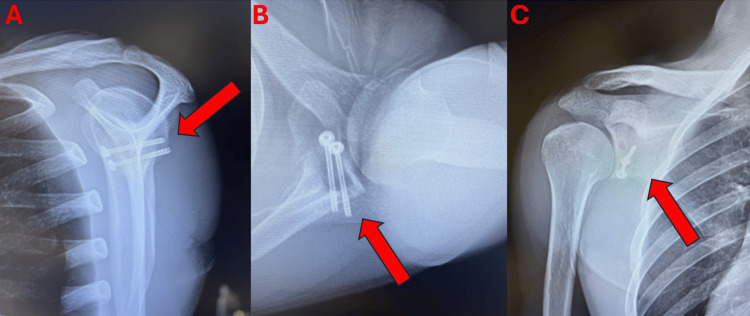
Postoperative radiographs of the right shoulder following revision anterior glenoid reconstruction with distal tibial allograft. Postoperative radiographic views of the right shoulder following distal tibial allograft reconstruction for revision anterior glenoid stabilization, demonstrating graft positioning and fixation in the posterior (A), axial (B), and anterior (C) orientations.
